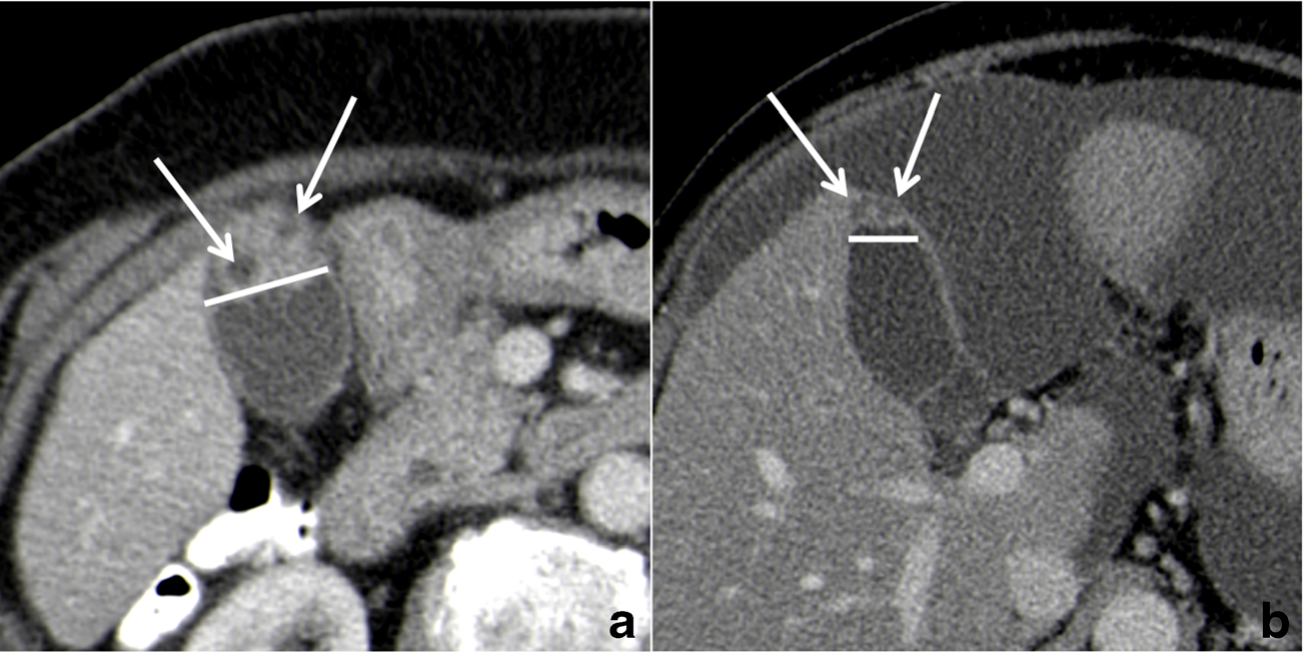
Gallbladder adenomyomatosis: typical CT findings. At CT, gallbladder adenomyomatosis is characterized by mural thickening (*line*) containing cystic spaces representing Rokitansky–Aschoff sinuses (*arrows*). Large RAS can be easily identified on 3-mm-thick reconstructions (a), whereas for identifying smaller RAS thin slices evaluation is crucial (b)
